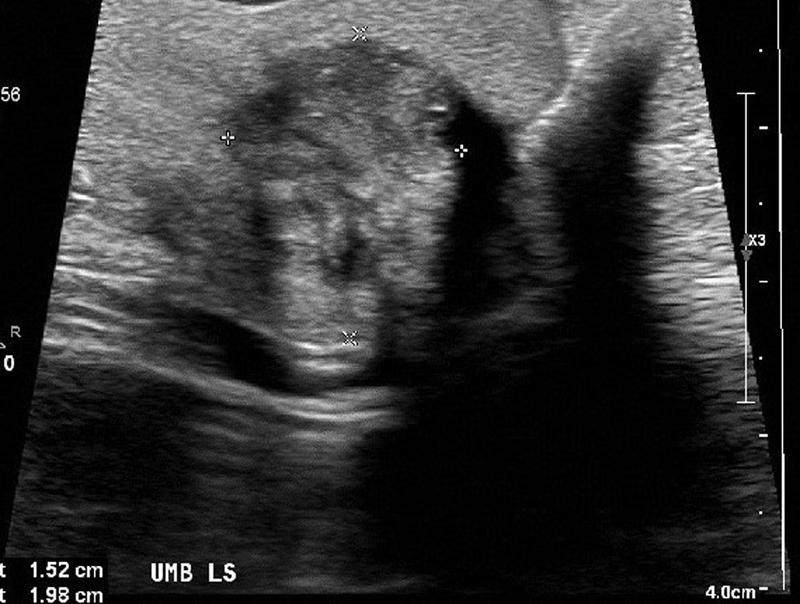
Ultrasonography of the umbilicus demonstrated a 2 × 1.5 cm heterogeneous echogenic material within the umbilicus.
